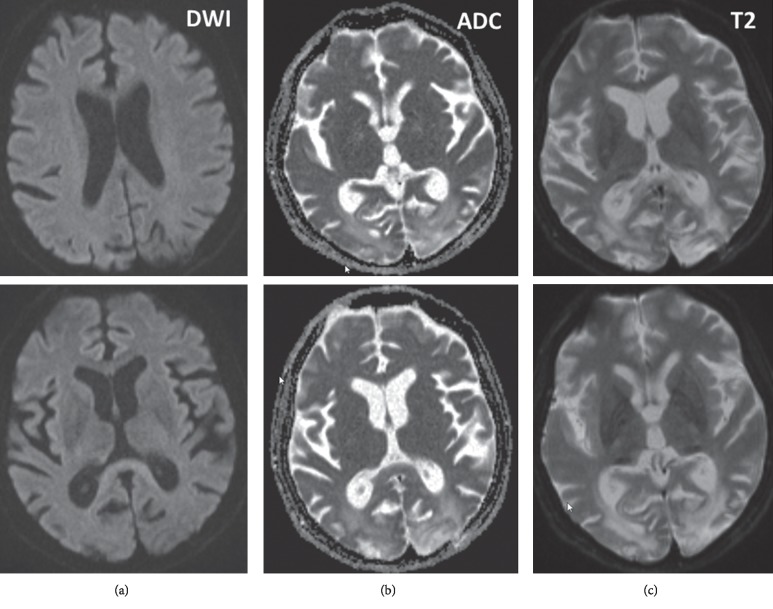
Cerebral MRI, 2 months after onset of psychosis, epilepsy, and visual impairment showing a slightly hyperintense right occipital lesion and a slightly hypointense similar lesion in the left occipital area, which were both hyperintense on ADC and hyperintense on T2-weighted images. Within the right occipital lesion, cortical and subcortical bleeding was identified. The occipital lesions were retrospectively interpreted as consecutive, subacute SLLs.
